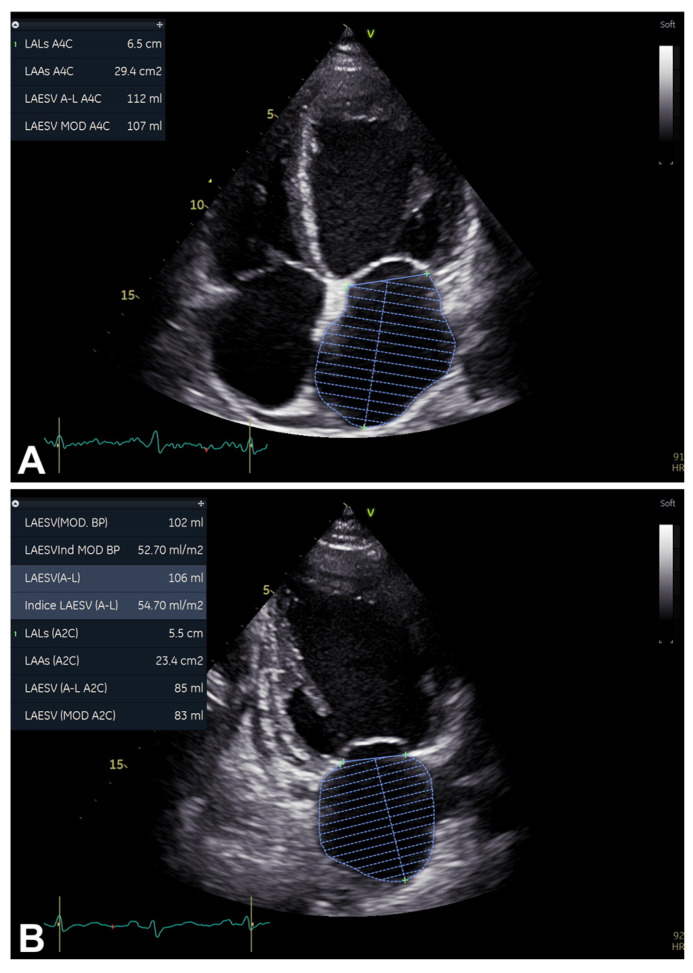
An example of LAVi evaluation, assessed from 2D apical four-chamber (A) and two-chamber (B) views. Note: 2D, two-dimensional; LAVi, left atrium/atrial volume index (measured in mL/m^2^) (see text, tables, and references).
